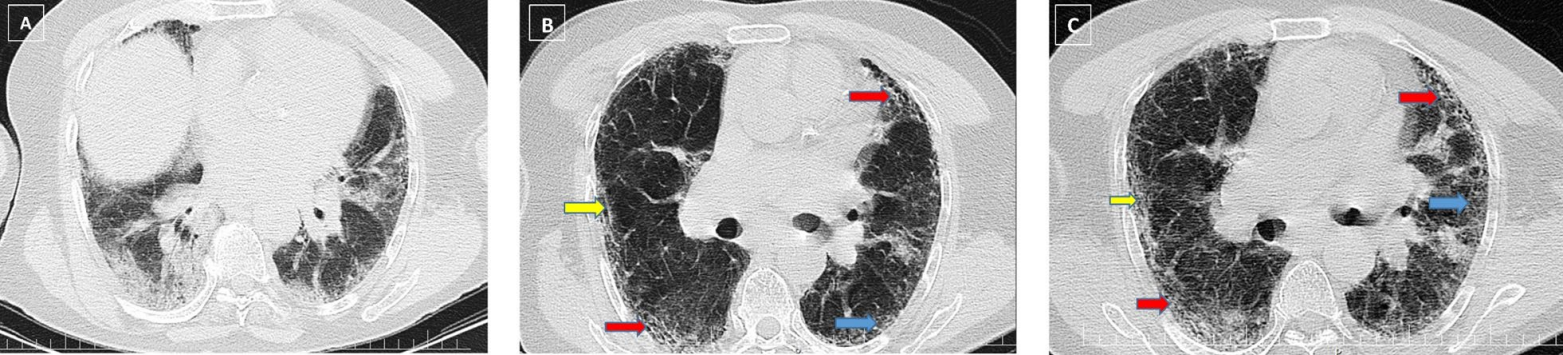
A 70-year-old male patient presented with fever, chills, cough, and myalgia, who was admitted to hospital and was discharged on day 18 of admission. Ground glass opacities and consolidations in multiple pulmonary lobes are observed in the initial CT scan (A). The total CT severity score was calculated as 21, and the disease severity was moderate. Three-month follow-up chest CT (B) shows parenchymal bands (yellow arrow) and interlobular septal thickening (blue arrow) and honeycombing (red arrow). Six-month follow-up chest CT (C) shows parenchymal bands (yellow arrow) and interlobular septal thickening (blue arrow) and honeycombing (red arrow) as well
